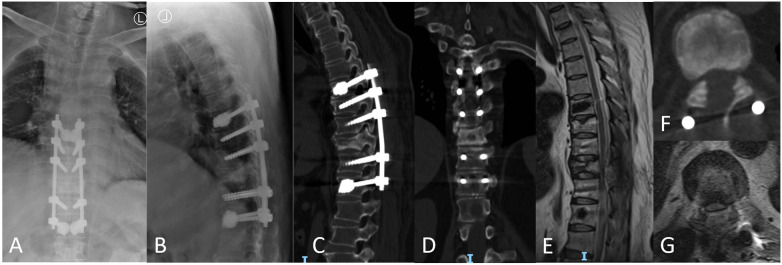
Postoperative imaging. Anteroposterior (A) and lateral (B) radiographs demonstrating stable pedicle screw fixation with bone cement augmentation at T7 and T12, maintaining proper thoracic alignment. Sagittal (C) and coronal (D) views of CT and MRI (E) scans demonstrating complete removal of the ossified ligamentum flavum at the T10-T11 level and adequate decompression of the spinal canal. Axial CT (F) and MRI (G) images confirming total excision of the ossified lesion and satisfactory decompression of the spinal cord.
